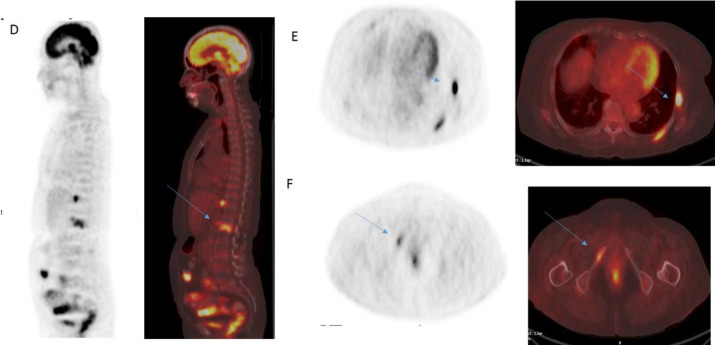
FDG PET (SUV range:0-4) and PET/CT fused sagittal image (D) revealed FDG avid lytic lesion involving L1 vertebral body [Arrow] and transaxial images revealed lytic lesions involving left sided ribs (E), and right inferior pubic ramus(F) [Arrow]
